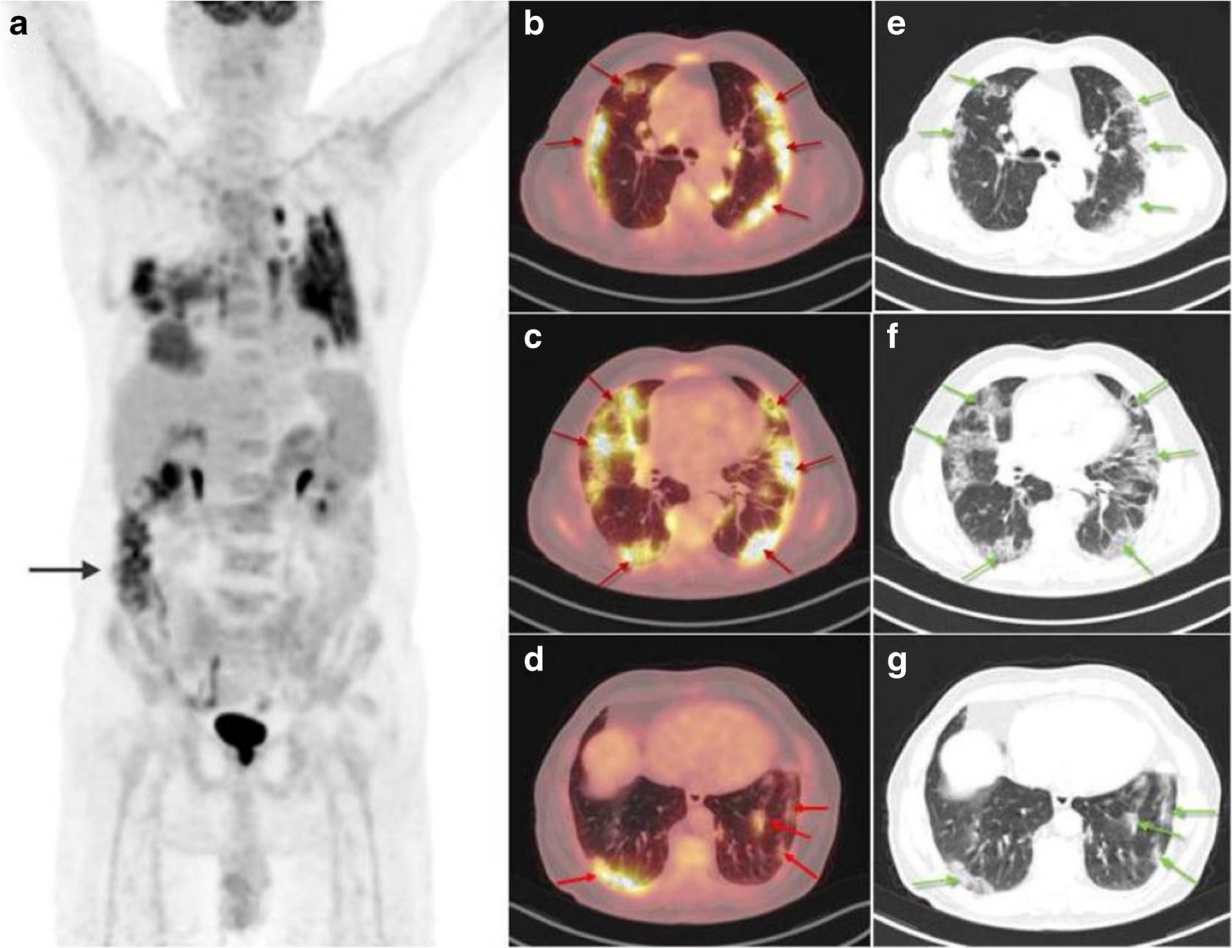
Incidental detection of COVID-19 in an 82-year-old patient with a history of adenocarcinoma of the colon. Increased ^18^F-FDG uptake (SUVmax 8.6) was observed in the bilateral lung fields (a, maximal intensity projection image; b–d, axial fused PET/CT images), corresponding to the bilateral ground-glass opacities on CT (e–g, axial CT images). Physiologic activity was also observed in the bowel indicated by a black arrow in the PET image (reproduced by permission from reference [27])
